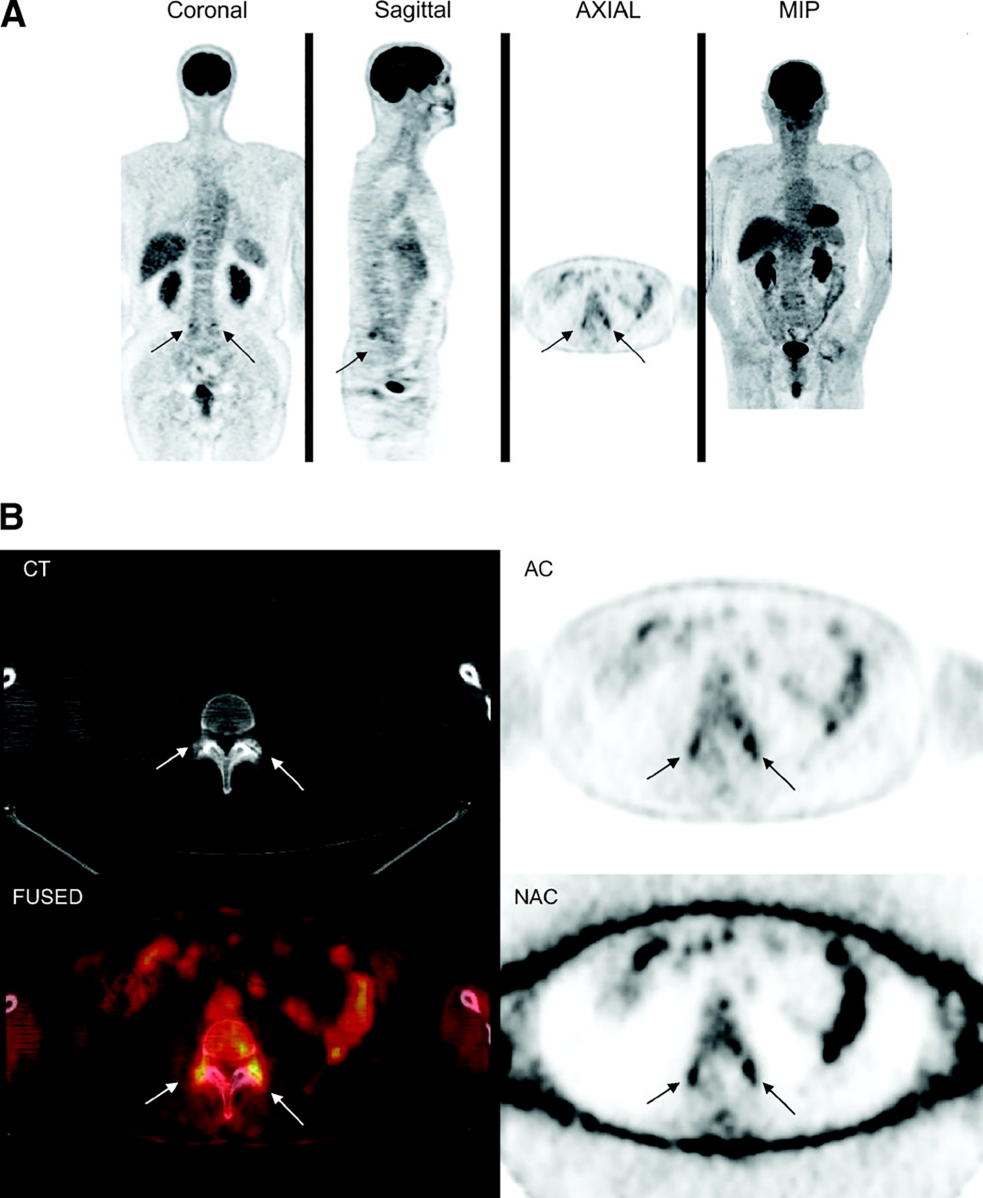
^18^F-FDG PET/CT images of lumbosacral spine show increased 18F-FDG uptake in region of facet joint, corresponding to abnormal findings on CT (arrows). (A) Coronal, sagittal, axial, and maximum-intensity-projection (MIP) PET images. (B) CT, attenuation-corrected, fused, and nonattenuation-corrected PET images. AC, attenuation-corrected PET image; FUSED, fused CT and PET images; NAC, nonattenuation-corrected PET image. This figure was originally published in *JNM*. Rosen RS, et al. J Nucl Med. 2006;47:1274–1280. © SNMMI. Reproduced with permission. (59).
